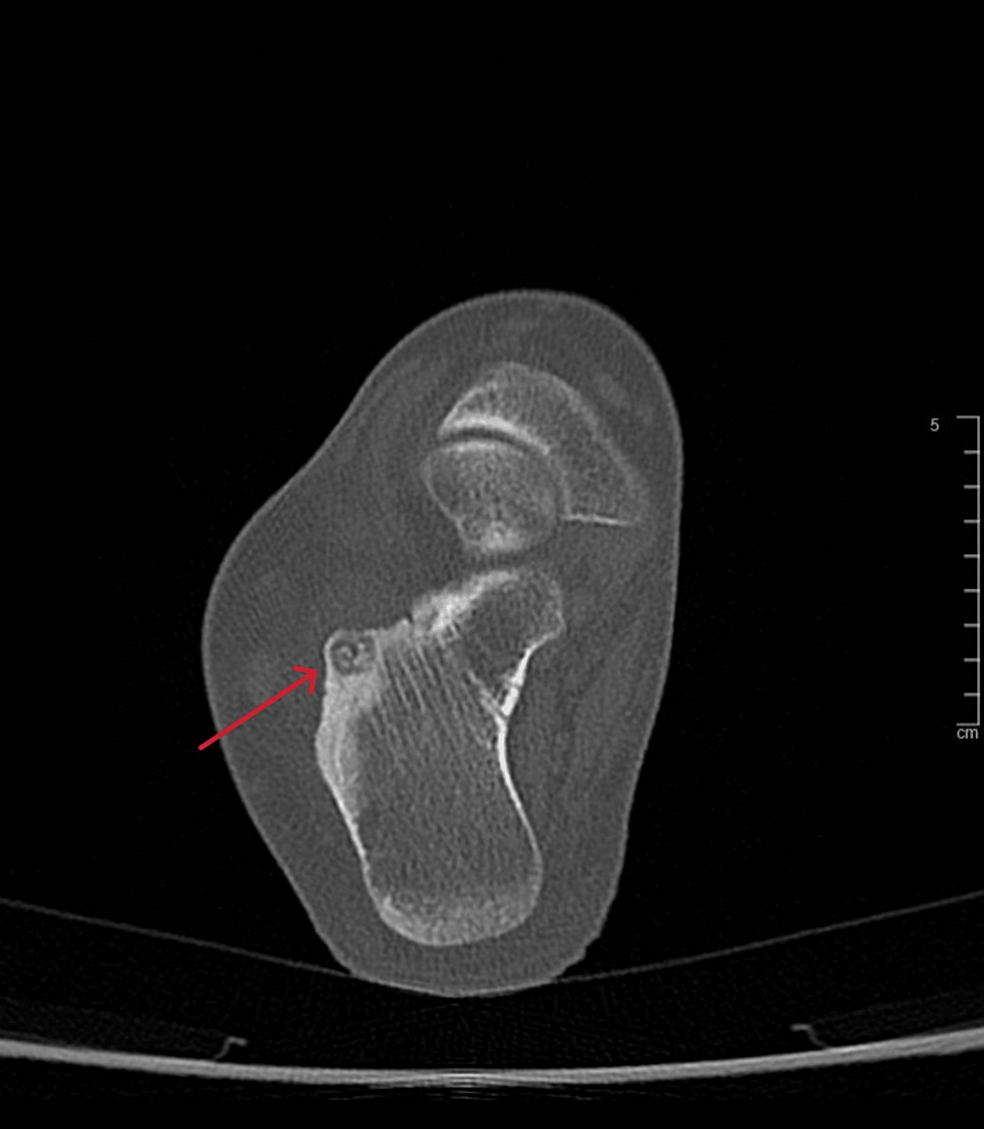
Axial view CT scan of the right ankle at the time of presentation CT: computed tomography
